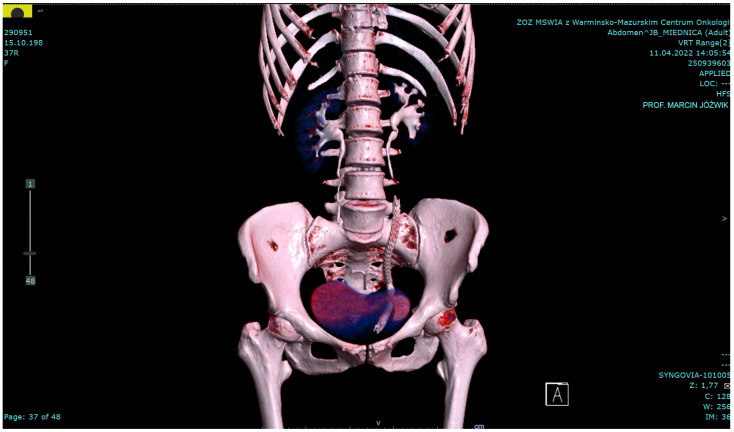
A postoperative CT scan showing the normal right ureter and a 20-centimeter-long allium stent in place in the left ureter and dilating it. Postoperatively, the stent migrated slightly into the urinary bladder, and its 5 cm end is visible in the lumen (11 April 2022).
